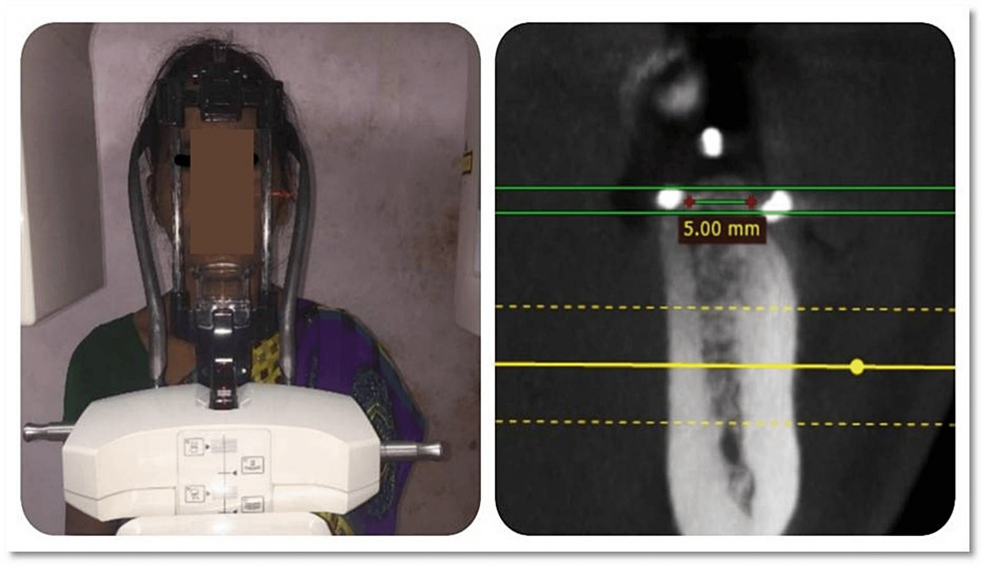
Alveolar ridge measurement by cone beam computerized tomography (CBCT)
